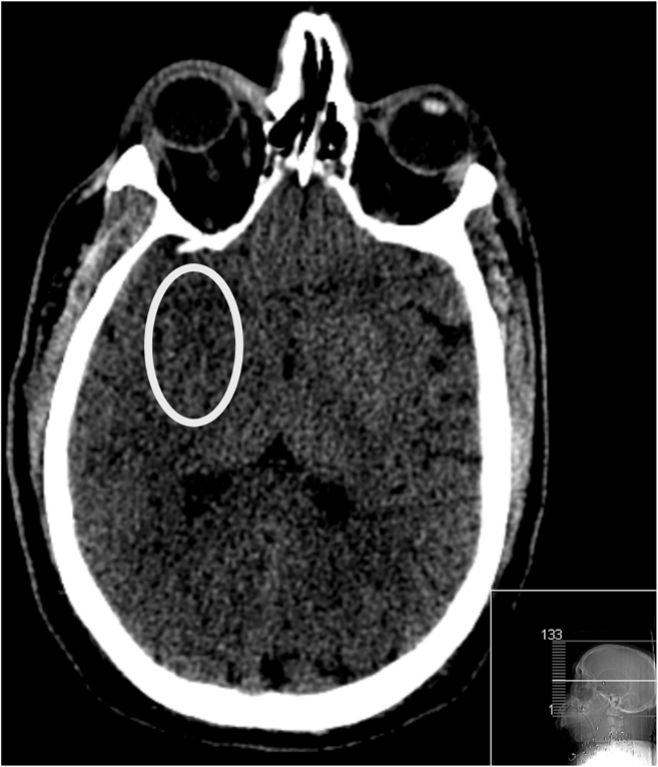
Axial image from non-contrast CT brain at presentation.
